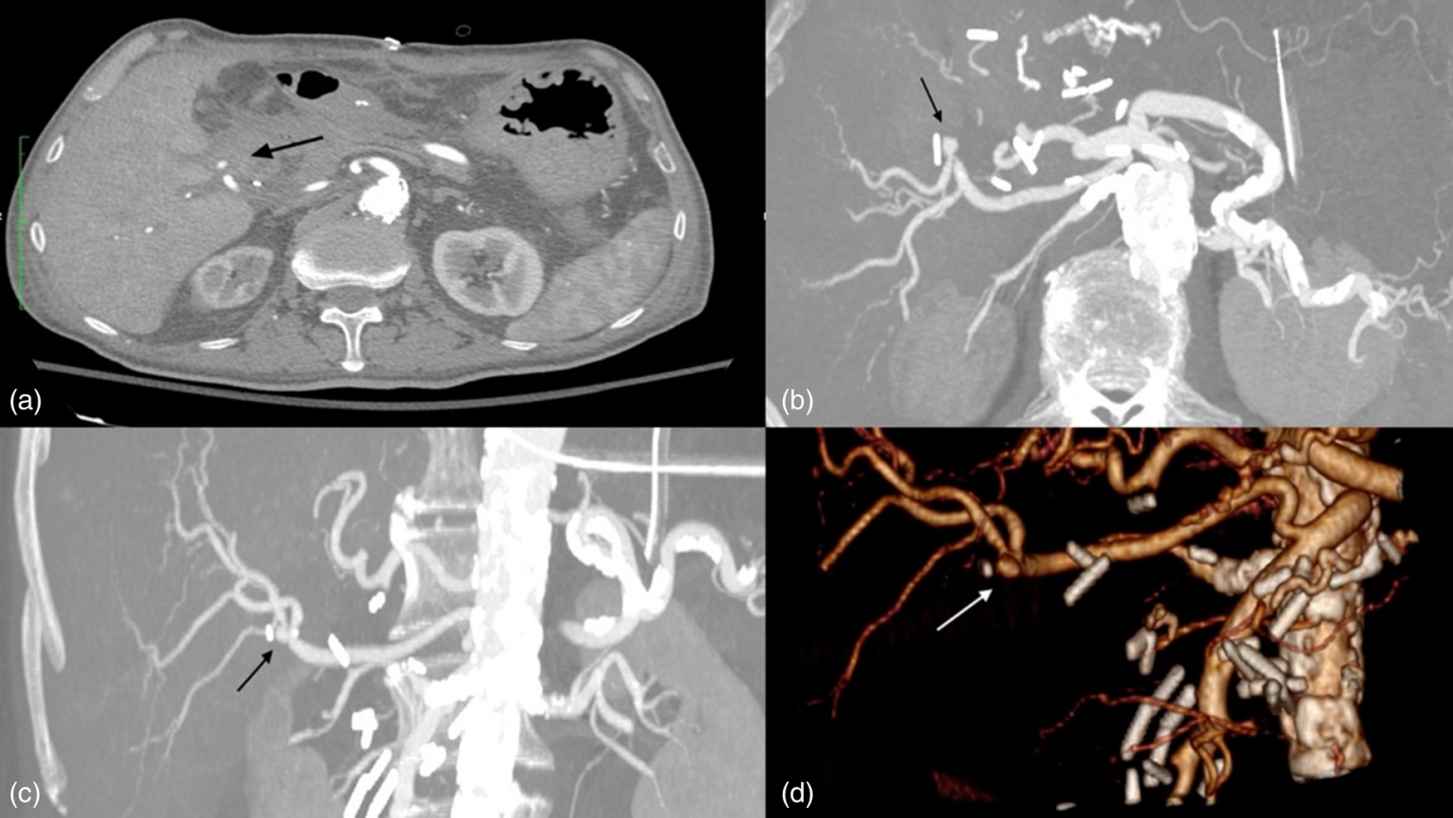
CTA. Arrows show hepatic artery pseudoaneurysm: (a) axial view, (b) axial MPR, (c) coronal MPR, (d) 3d reconstruction.
